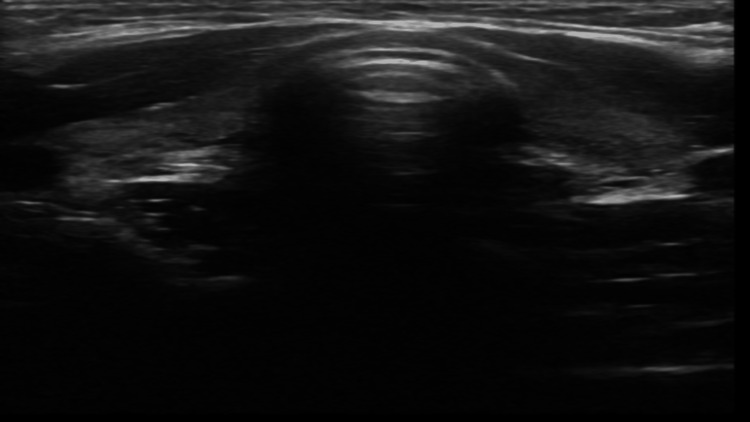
Thyroid ultrasound demonstrating bilaterally small, heterogeneous thyroid glands without focal nodules or increased vascularity, findings consistent with chronic autoimmune thyroiditis.
